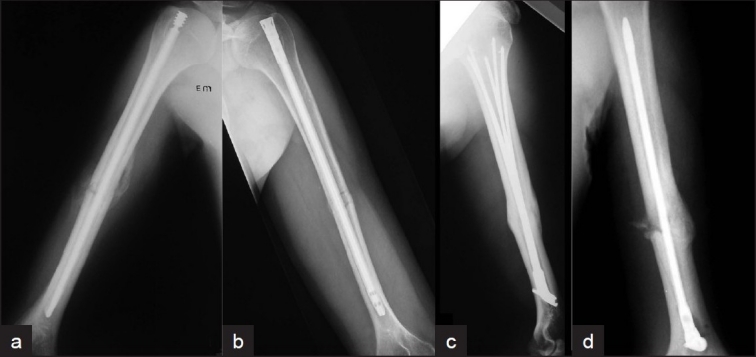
Plain radiographs of arm (anteroposterior a, b and lateral c, d views) of “Bio” nails showing (a) True-Flex (Encore), (b) Garnavos antegrade (MERETE), (c) Marchetti (Zimmer), (d) Garnavos retrograde (MERETE)
